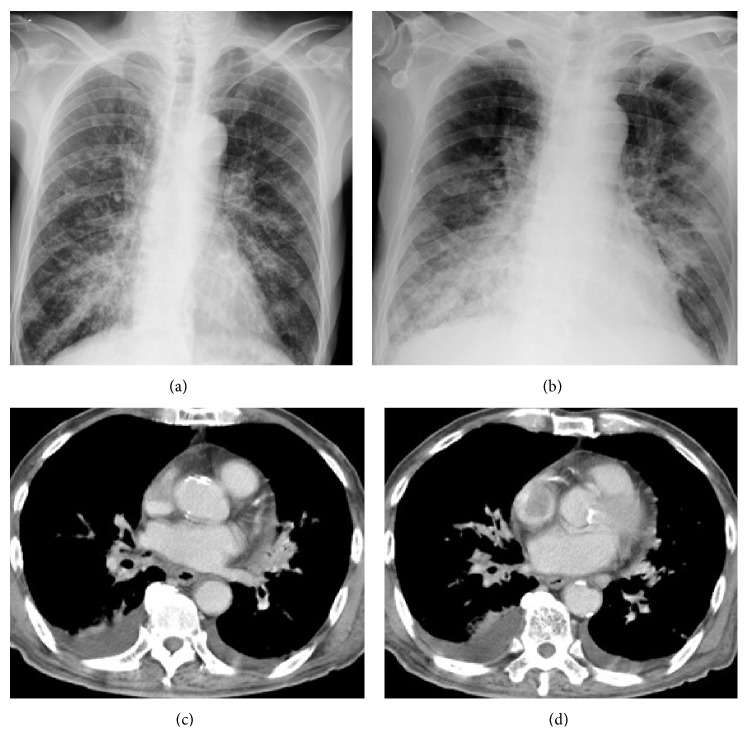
Pulmonary vein obstructive syndrome (PVOS). A 78-year-old man with rectal cancer. Chest X-ray (a) before and (b) at the onset of PVOS showed right low lung and pulmonary hilum increase haziness. CT scan ((c) and (d)) revealed tumor/thrombosis located in the bilateral superior and inferior pulmonary veins.
